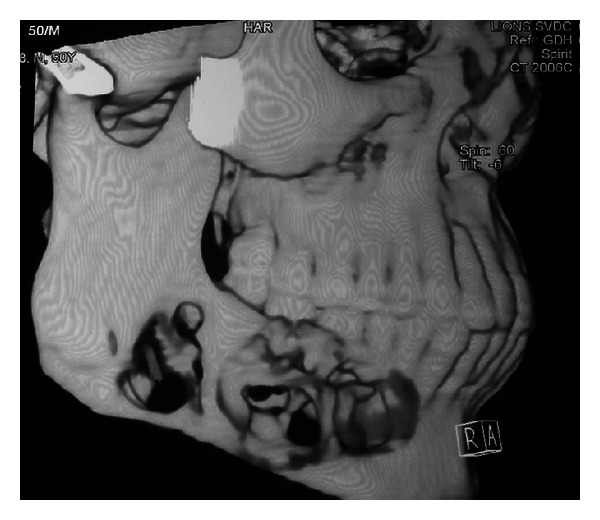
3D reformatted CT scan showing perforation of the mandible from 44 to angle region on right side.
